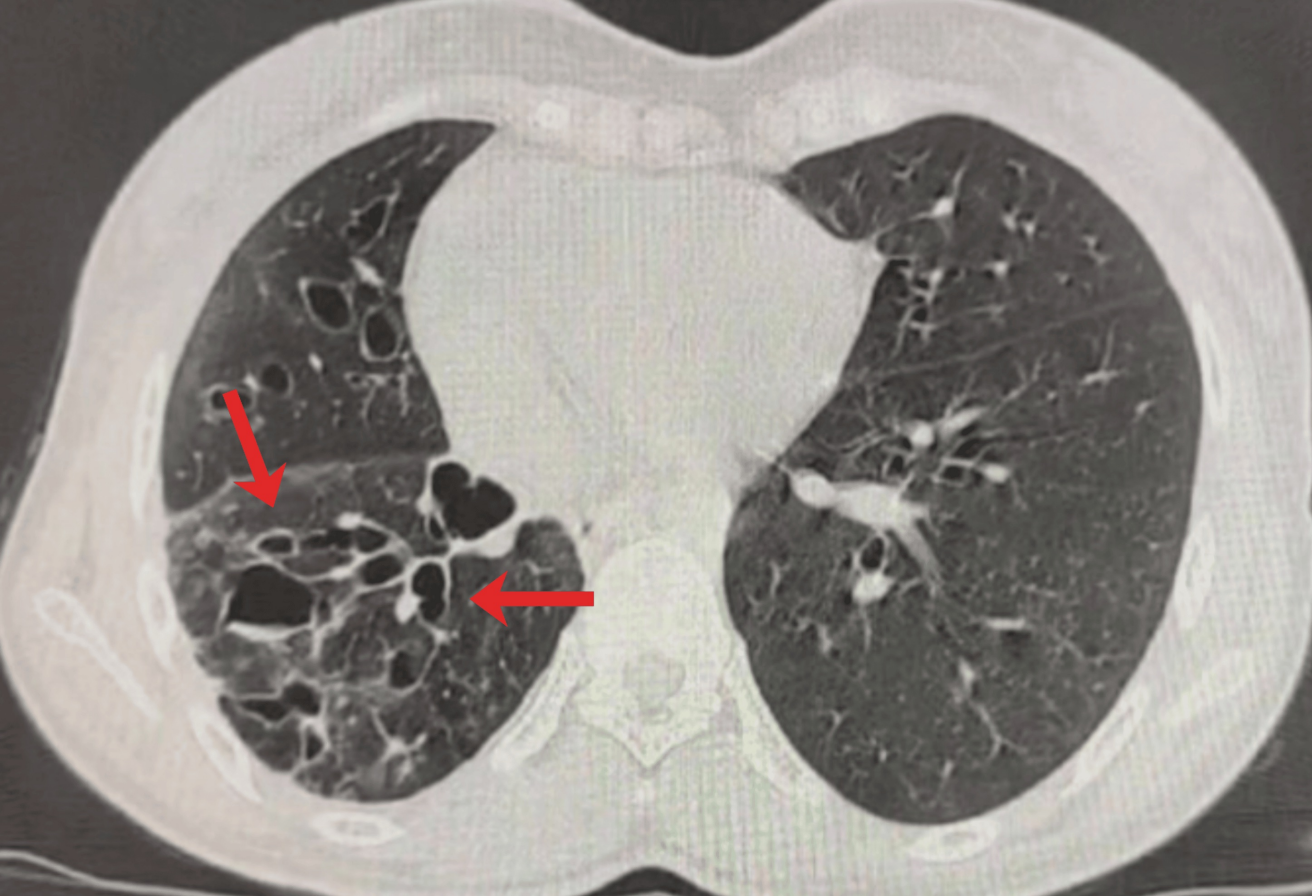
Axial HRCT chest (lung window) demonstrating cystic and varicose bronchiectatic changes (arrows) in the right middle and lower lobes, with mucus plugging and bilateral mosaic attenuation consistent with post-obstructive bronchiectasis secondary to tracheobronchial luminal obstruction. HRCT: high-resolution computed tomography
